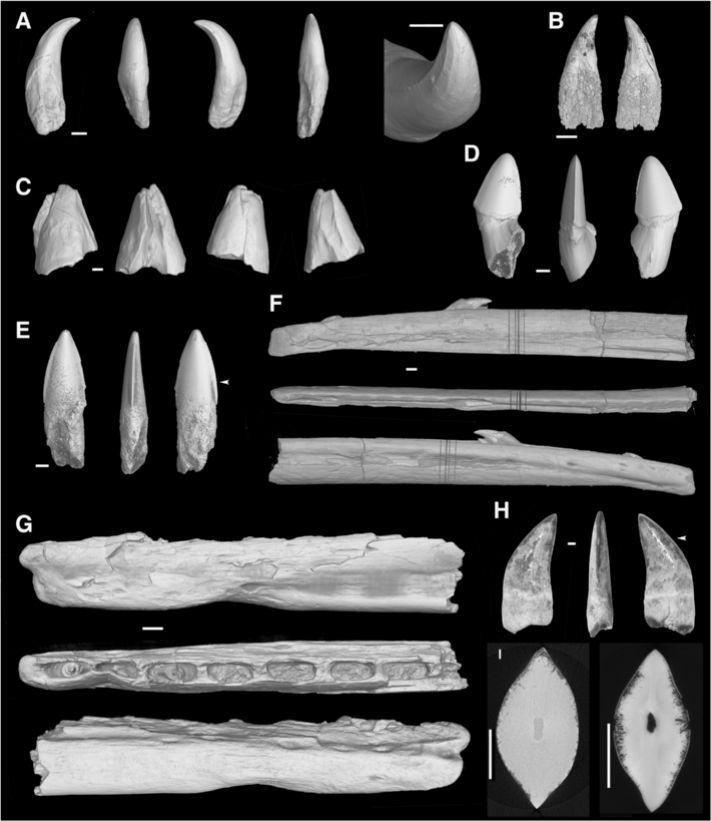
X-ray microtomographic views of the studied teeth or dentary fragments with teeth of *Hesperornis regalis*, *Ichthyornis dispar*, and Ichthyornithiformes indet. a Isolated tooth of *Hesperornis regalis* YPM.1206B, complete tooth. From left to right: lingual, mesial, labial and distal views, and apical part showing fluted ornamentation. b Virtually extracted tooth TH_2_ from *Hesperornis regalis* dentary YPM.1206A, lingual (left) and labial (right) views. Isolated teeth of *Ichthyornis dispar*: c YPM.1460, damaged tooth tip fragment, four views; d UAM_PV93.2.133_1, nearly complete tooth, from left to right: lingual, mesial and labial views; e UAM_PV93.2.133_2, nearly complete tooth, from left to right: lingual, mesial and labial views (small arrow shows level of horizontal section in i). Incomplete dentaries of: f
